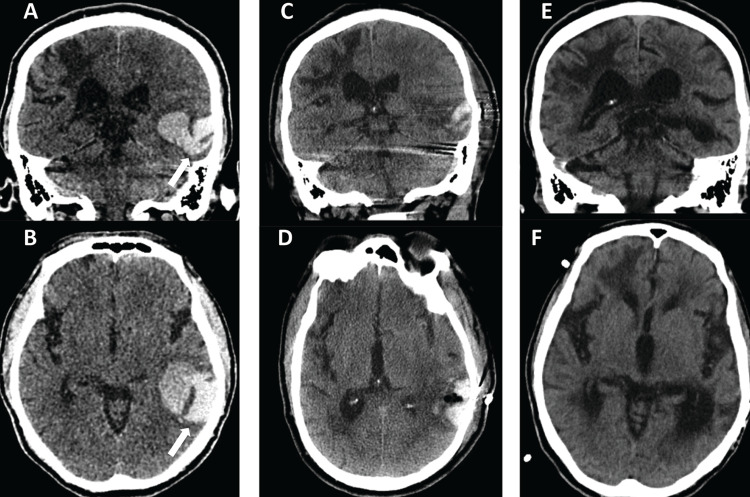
Case 2 - second admission pre- and postprocedure and follow-up imaging. Preprocedure second admission non-contrast coronal (A) and axial (B) head computed tomography (CT) demonstrated a 37.8 mL left posterior temporal hemorrhage (arrow). Postprocedure coronal (C) and axial (D) non-contrast CT demonstrated a 76.7% evacuation rate and the remaining hematoma measuring 8.8 mL. Coronal (E) and axial (F) follow-up imaging more than one year after the second hemorrhage showed the expected evolution of gliosis and encephalomalacia with hemorrhage resolution.
